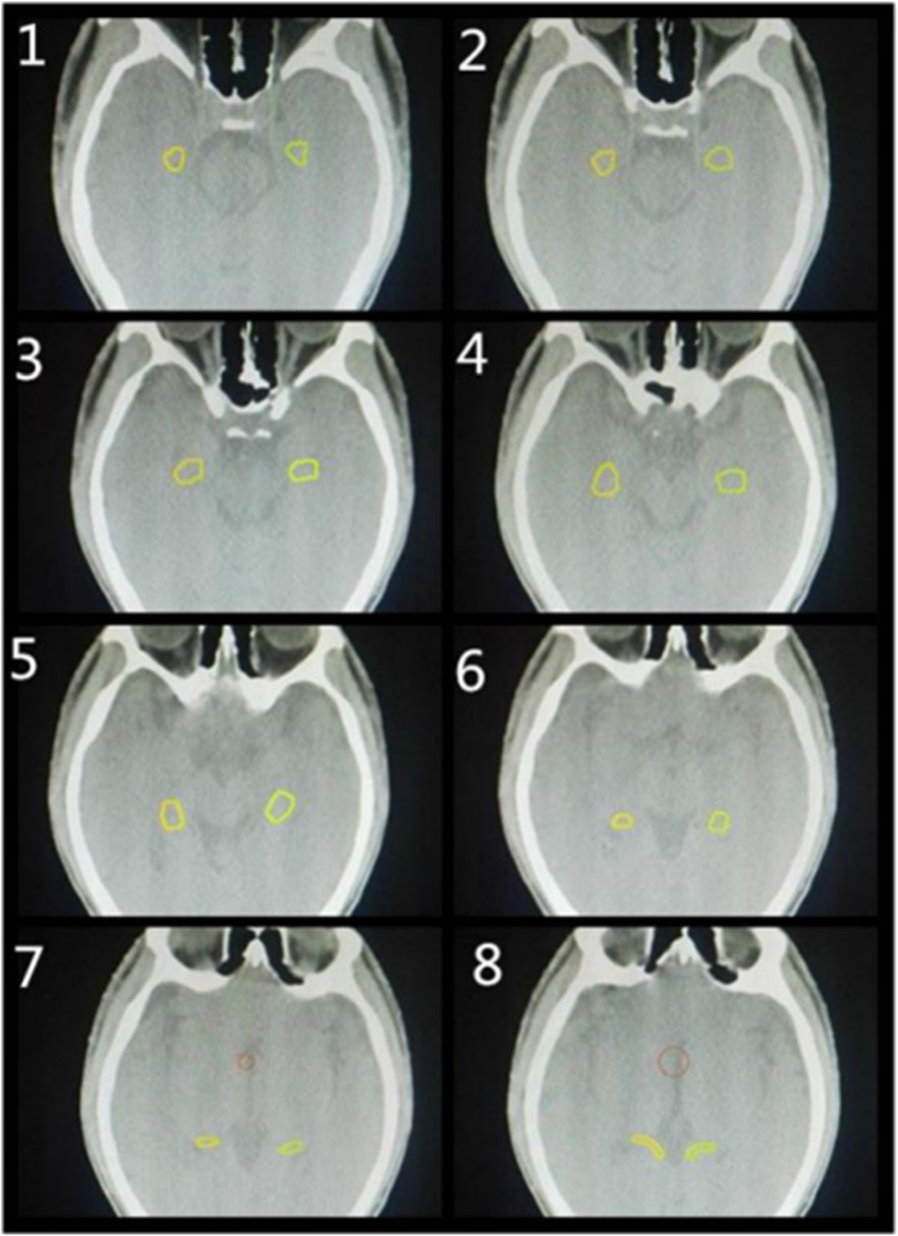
Axial images of the hippocampus contour based on the MRI-CT images fusion
